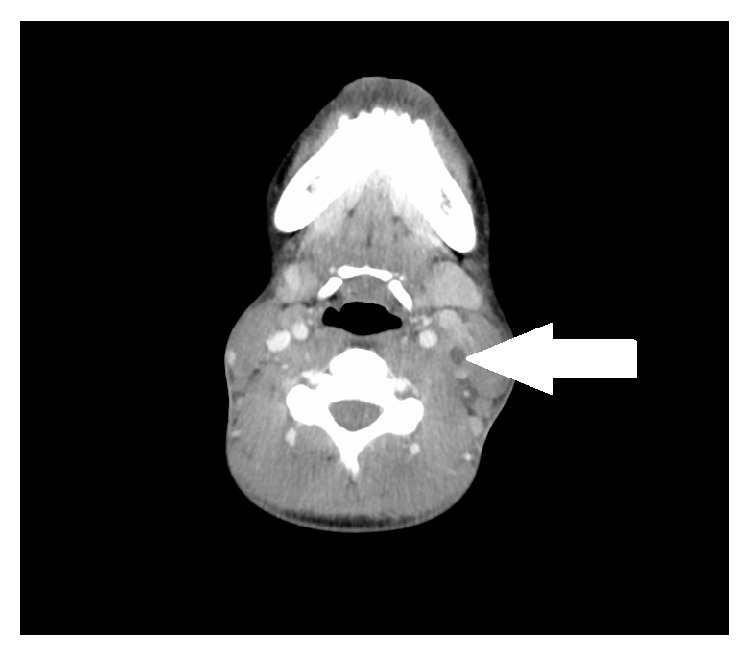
Contrast CT scan of the neck showing a thrombus in the left jugular vein (arrow).
